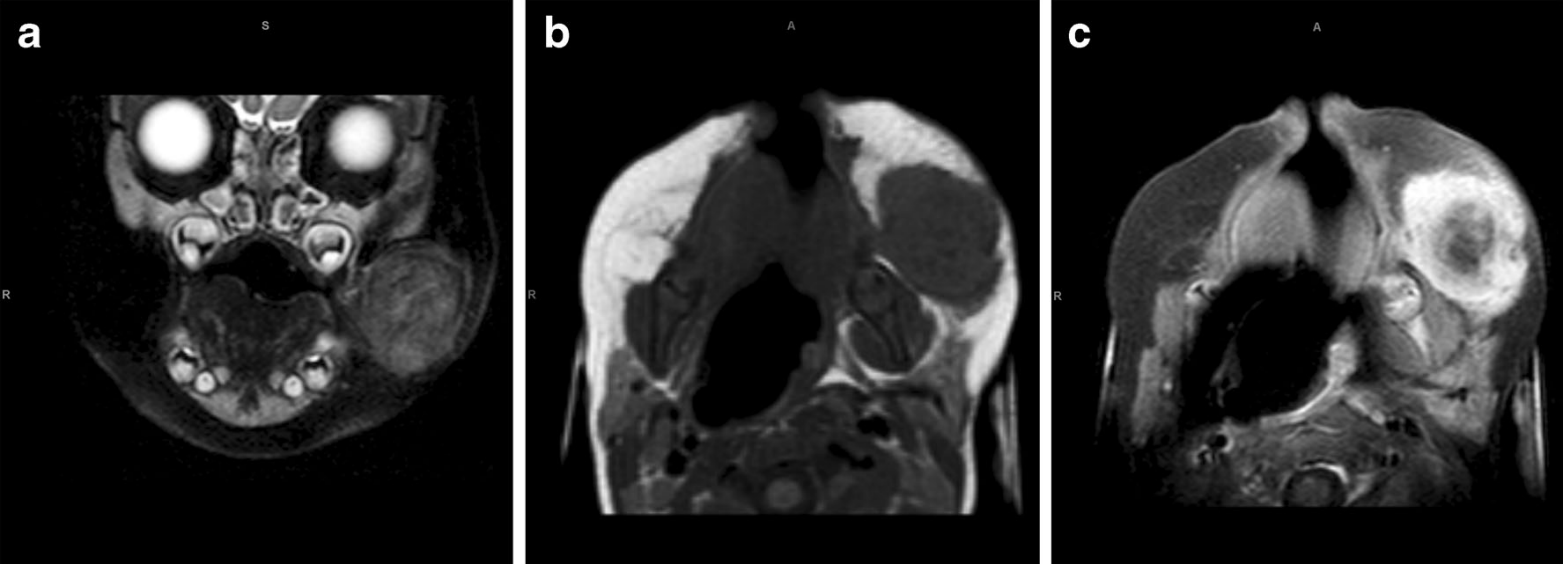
Infantile fibrosarcoma. Coronal T2-W (a) and axial T1-W (b) MRI images show a subcutaneous left masticator space lesion. The mass is avidly enhancing post-contrast (c) and contains foci of low signal consistent with central necrosis
